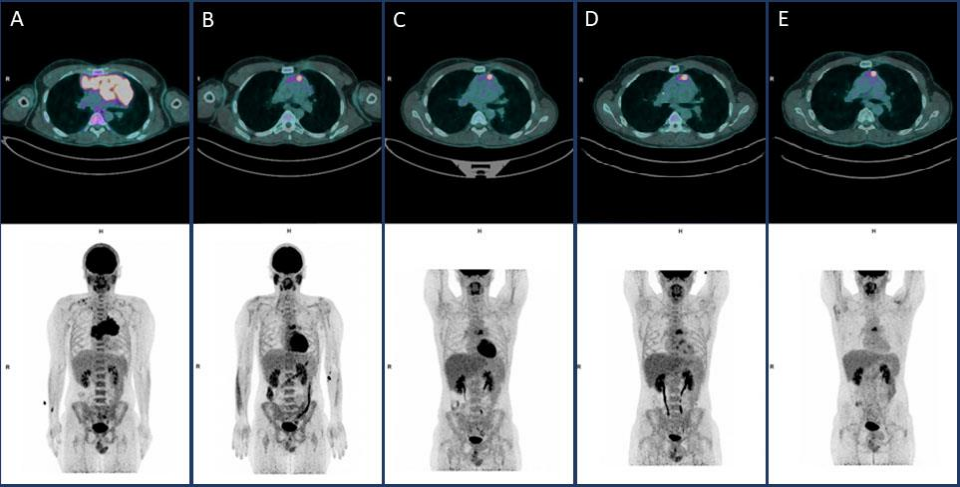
FDG-PET findings at [A] Baseline FDG-PET, [B] Interim FDG-PET (ABVD), [C] EoT FDG-PET (ABVD), [D], after three cycles BeGEV FDG-PET and [E] Follow Up after three months from last chemotherapy
